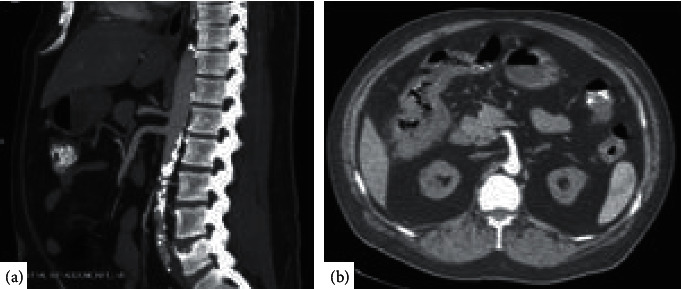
Computed tomography angiogram with contrast of the abdomen and pelvis demonstrating atherosclerotic disease of the abdominal aorta without involvement of major arterial supply of the intestine (a) and pericolic fat stranding with wall thickening of the right hepatic flexure (b).
